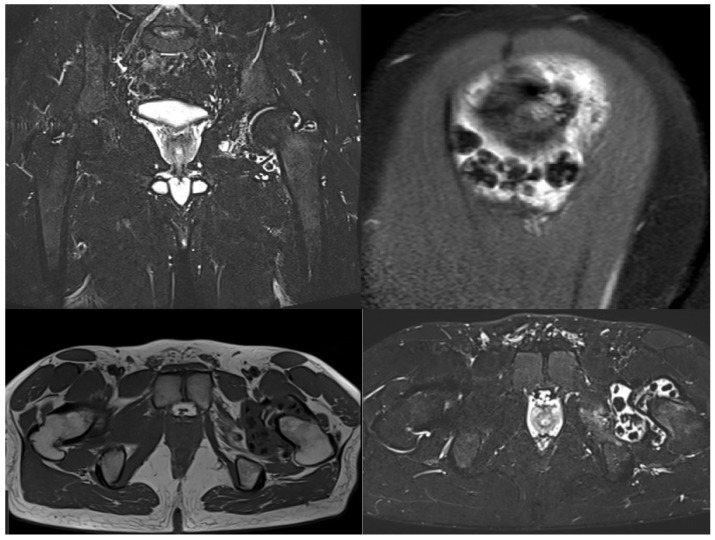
Coronal and axial MRI sequences show diffuse nodular synovial proliferation with numerous intra-articular loose bodies, partially calcified, exhibiting heterogeneous signal intensity and associated reactive changes.
